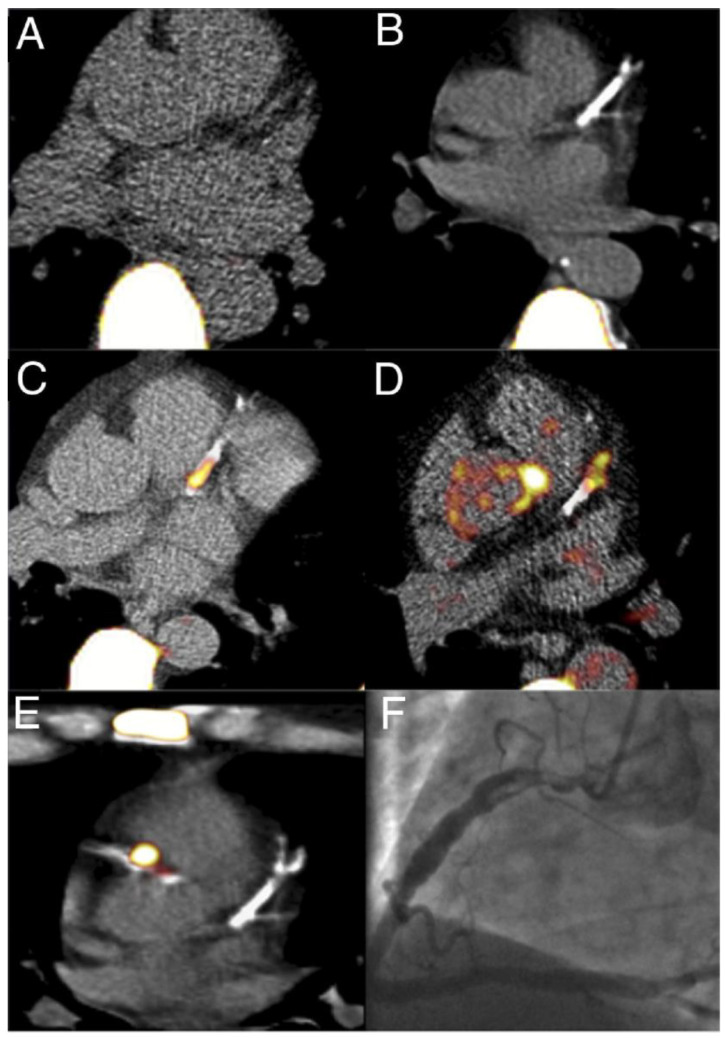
^18^F NaF PET/CT of coronary arteries demonstrating normal (no CT calcification and no PET ^18^F NaF accumulation) (A), macrocalcification on CT with no PET ^18^F NaF accumulation (stable plaque) (B), intense focal ^18^F NaF accumulation with corresponding CT calcification (C,D), intense focal accumulation of ^18^F NaF with more expansive CT calcification in a culprit lesion (E) with corresponding angiogram showing in situ thrombus and ulcerated plaque (F). Adapted from Dweck et al. [18]. 2017 Copyright the Authors.
